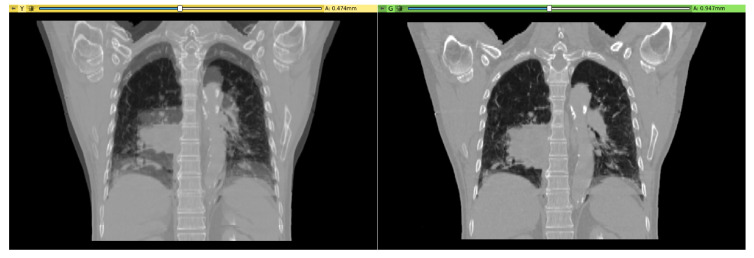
Computed tomography (CT) scans shown in overlay mode. In the left panel, before registration, it is possible to see the misalignment along the z axis. In the right panel, after linear registration, the displacement was accurately recovered.
